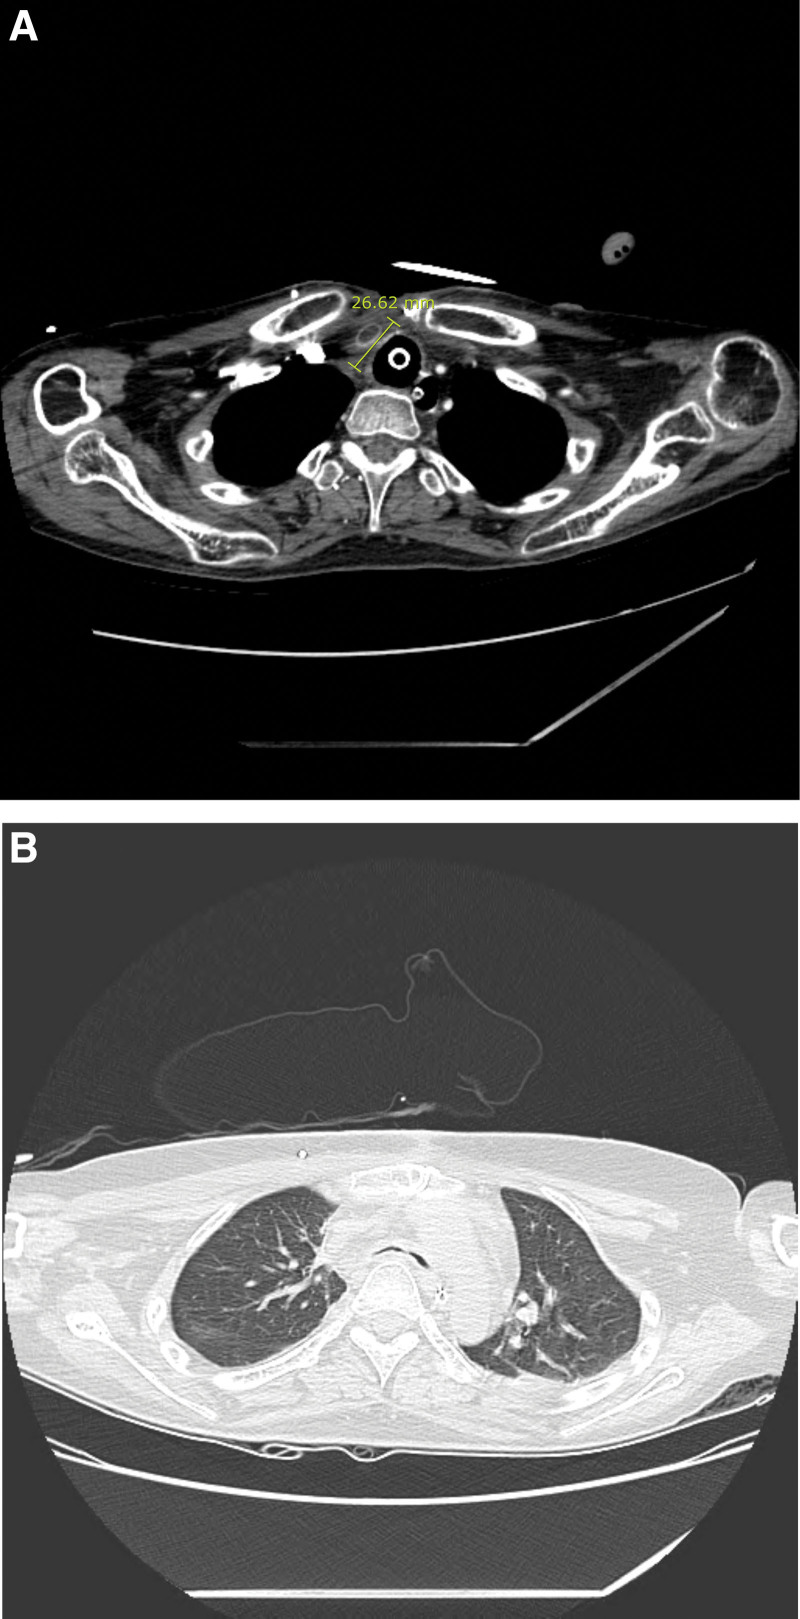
(A and B) Preoperative chest CT revealing diffuse tracheal wall thickening and advanced luminal narrowing of the trachea.
